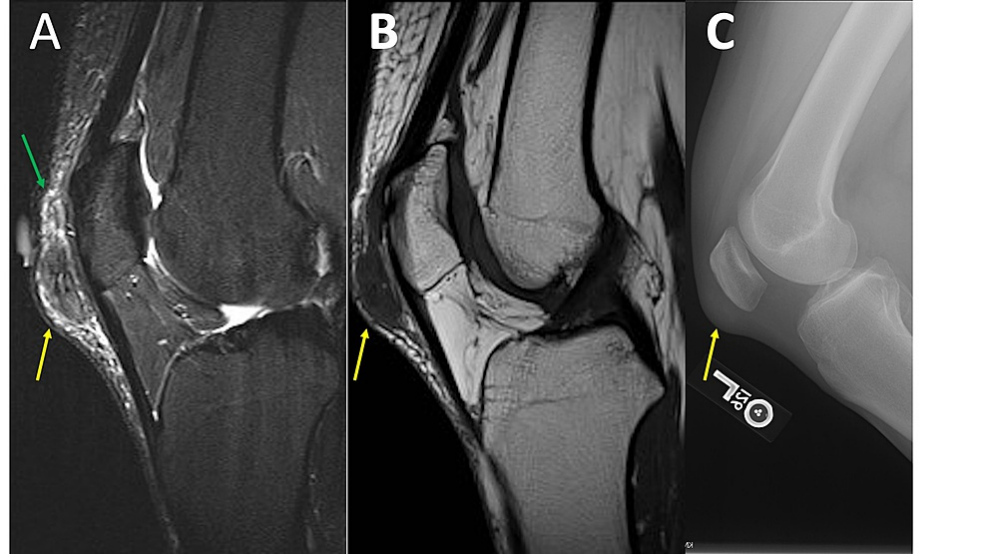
Gout MRI sagittal T2 fat saturation (FS) (3A) and MRI sagittal T1 (3B) demonstrate a nonspecific heterogeneous, predominately intermediate signal intensity soft tissue mass (yellow arrow) with surrounding soft tissue edema (green arrow) superficial to the patella and patellar tendon. Lateral knee radiograph (3C) demonstrates an area of soft tissue prominence (yellow arrow) corresponding to the mass noted on MRI. The mass appears slightly dense but there are no associated mineralized bodies, which would be a helpful discriminator. Also, no erosive changes are present. It is also important to recognize that the patella and patellar tendon are common sites for tophus formation. A search of the patient’s radiology records demonstrated findings suggestive of gout involving the foot (not shown).
